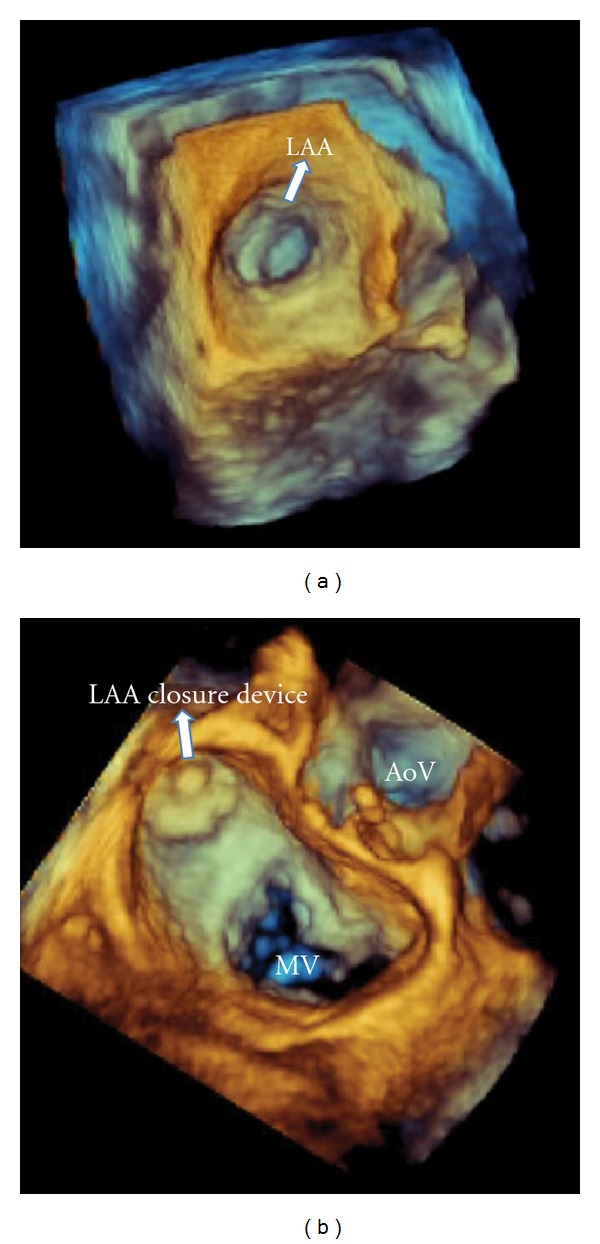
Real-time three-dimensional transoesophageal echocardiographic imaging of the left atrial appendage as seen from the left atrial perspective. (a) Preprocedural view of LAA. (b) LAA closure device deployed. (LAA: left atrial appendage; AoV; aortic valve; MV; mitral valve).
